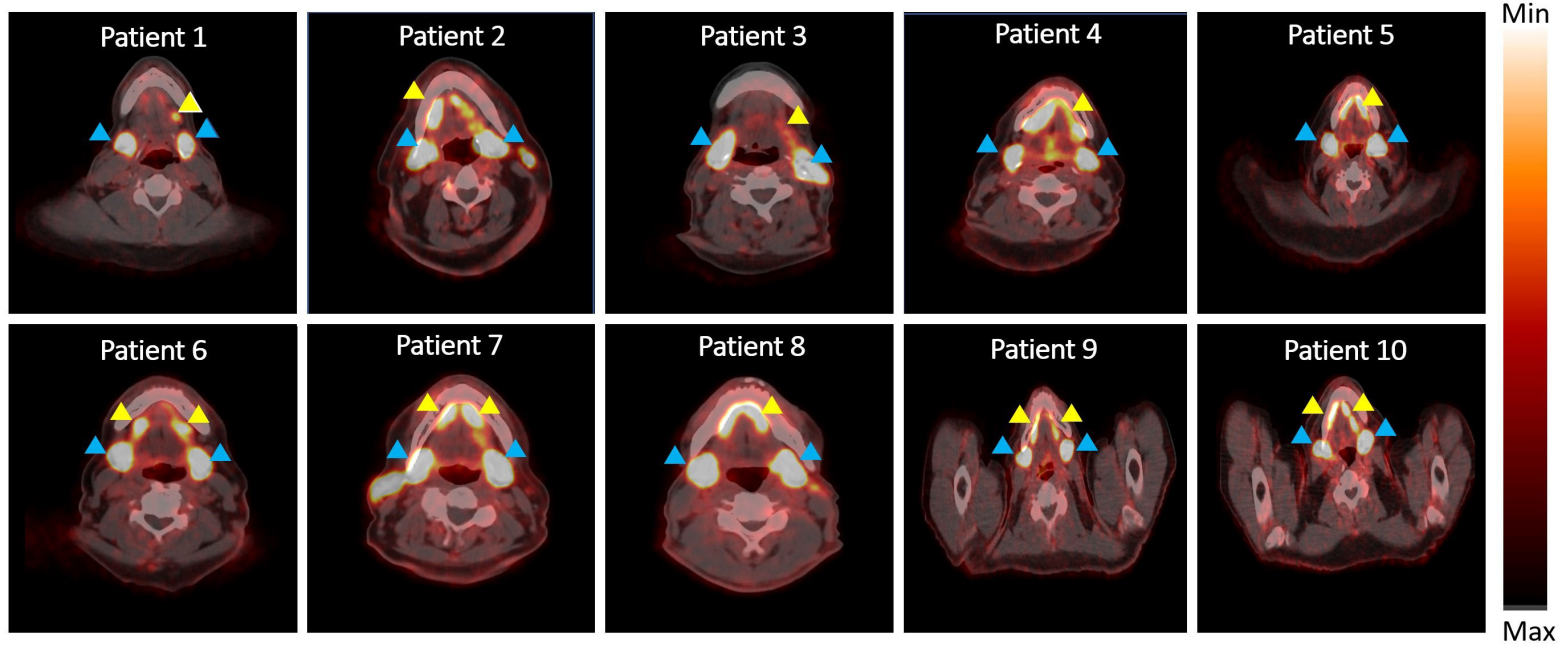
PET/CT scan showing radioactivity in salivary glands and saliva. Blue arrowhead indicates radioactivity accumulation in salivary gland and yellow arrowhead indicates radioactivity in saliva.
